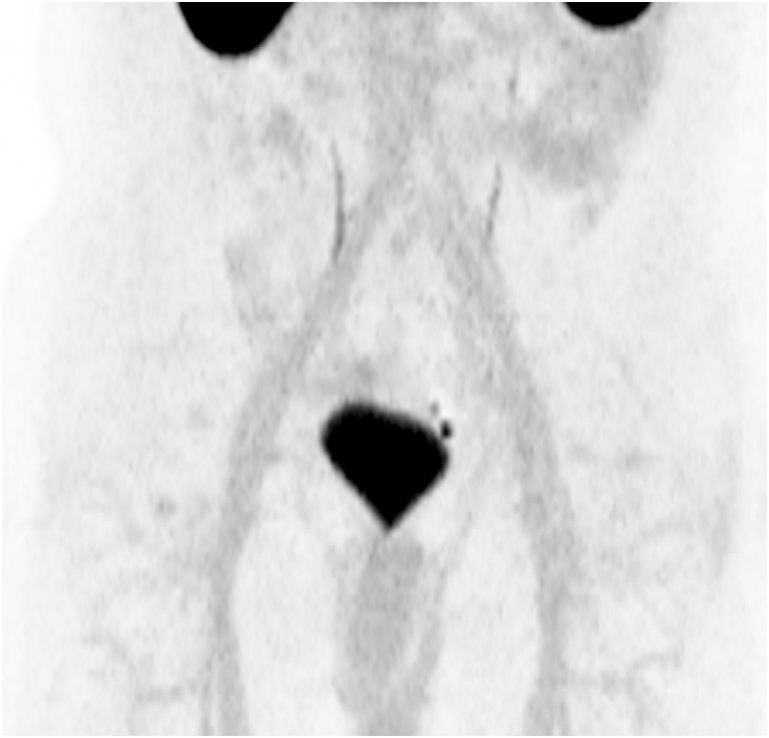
Patient with visible ureters and two pelvic lymph nodes. The figure presents the MIP image (SUV scale 0–10) of a patient with two visible ureters and two separately identifiable left pelvic lymph node metastases
